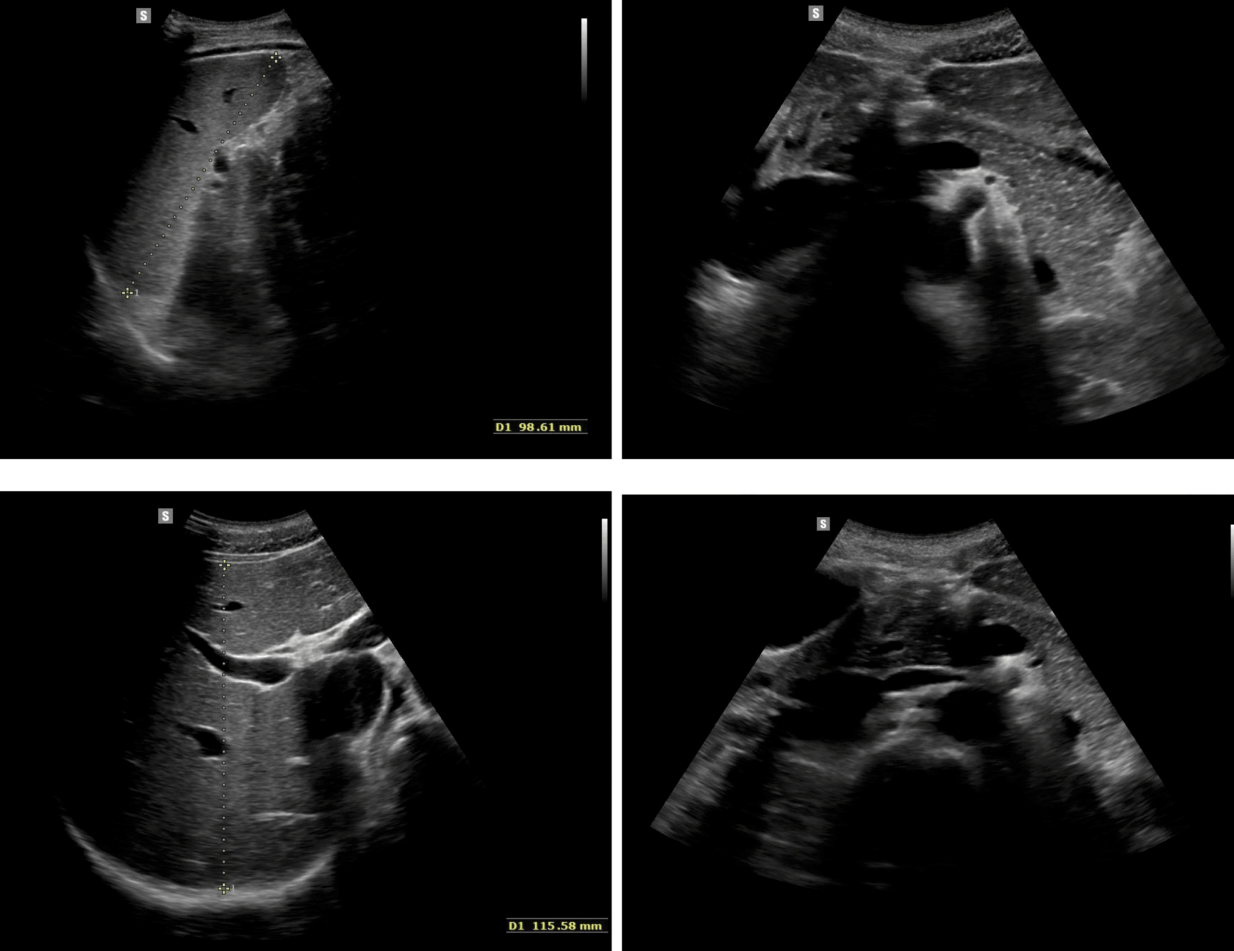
Ultrasound images of the abdomen Upper Right Image – Spleen: The spleen is of normal size (bipolar measurement: 99 mm) and exhibits a homogeneous echotexture without focal lesions. Lower Left Image – Liver: The liver is not enlarged (right lobe AP dimension: 116 mm), with a homogeneous structure and normal echogenicity. No focal lesions are present. The portal vein is of normal width. Upper and Lower Right Images – Upper Abdominal Structures: This view demonstrates normal-appearing upper abdominal structures. The pancreas appears normal in size and echotexture, with no focal lesions. The Wirsung duct is not dilated. No enlarged lymph nodes or abnormal retroperitoneal findings are observed.
